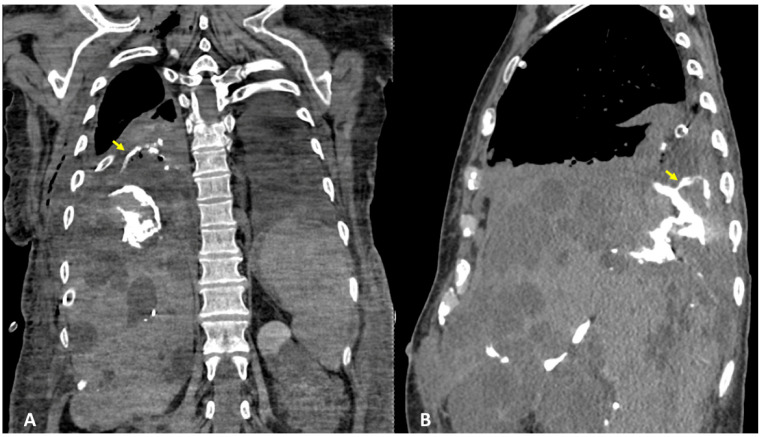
(A,B) CT performed with diluted iodinated contrast agent administered through the naso-biliar tube to obtain an exclusive enhancement of the intrahepatic biliary tract, and coronal (A) and sagittal planes (B). CT confirmed the presence of bile in a subcapsular intrahepatic cyst, showing the direct fistula with the right pleural cavity (arrows).
